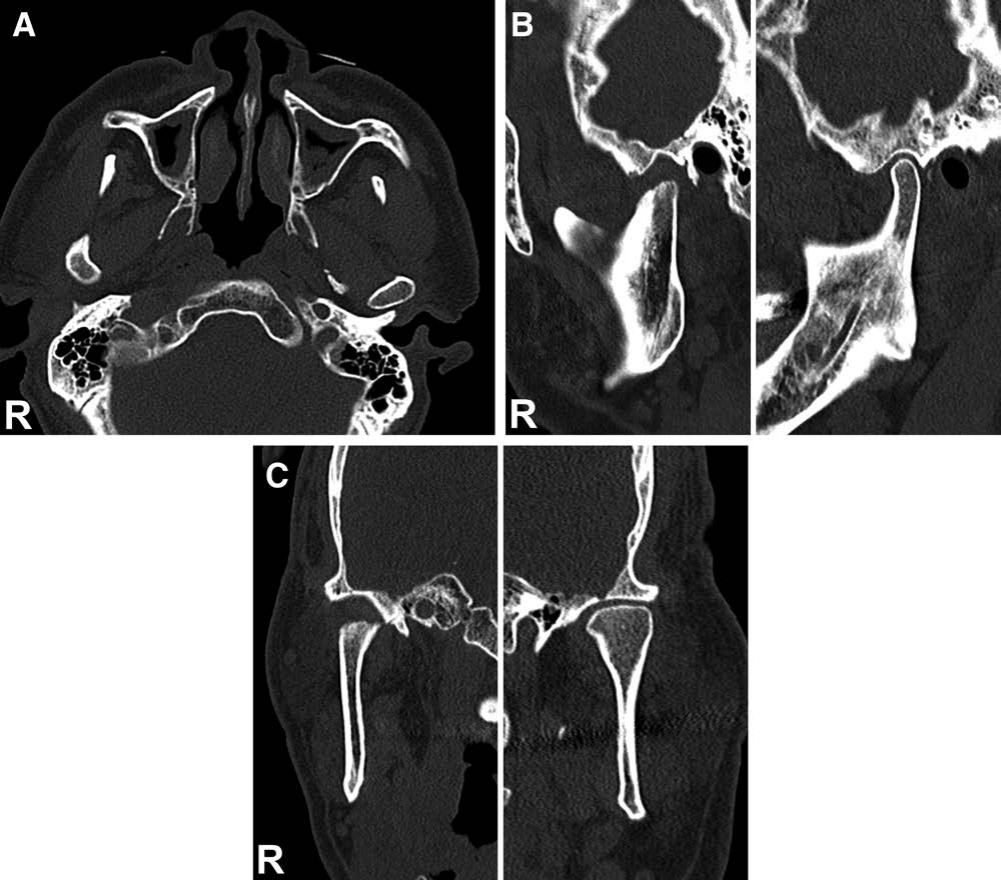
Six months postoperative follow-up CT imaging. (A) Axial view, showing some recortication of the resected area. (B) Oblique sagittal view, showing the remodeling in progress. (C) Coronal view, showing no signs of recurrence. CT = computed tomography.
